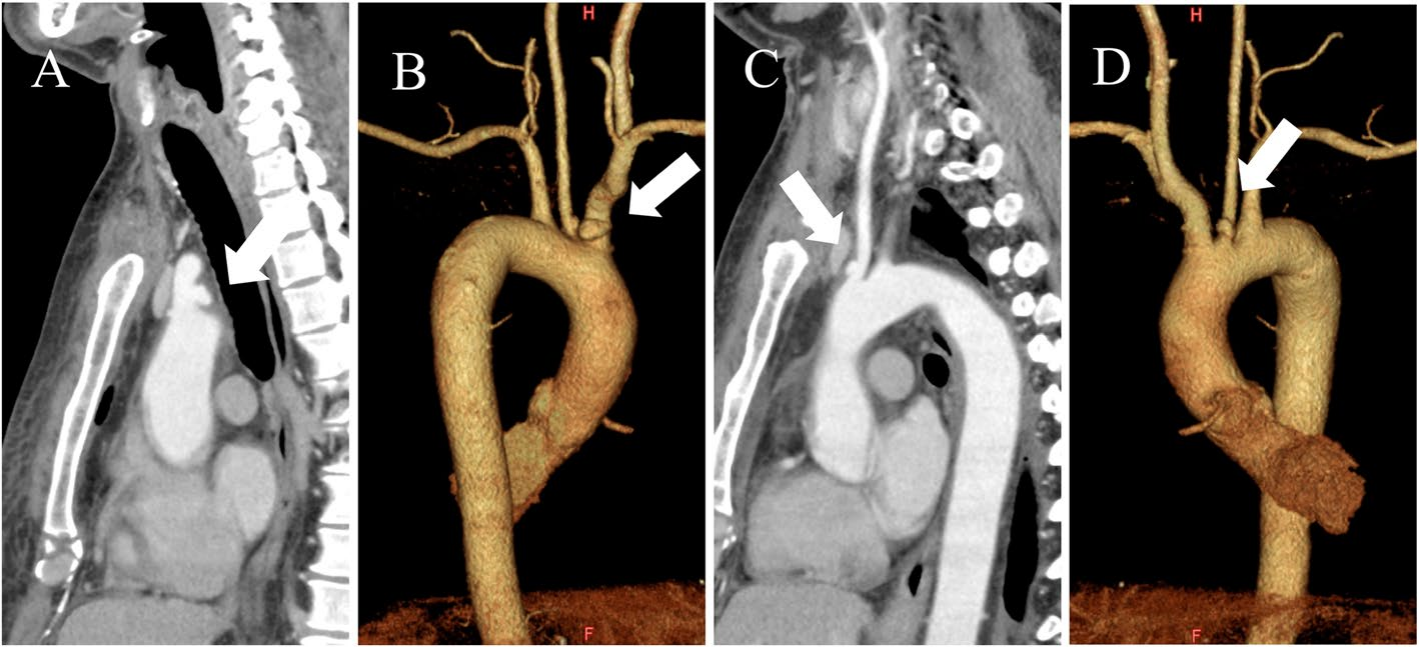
CT scans 2 weeks later following emergency admission. An enlargement of the BCA pseudoaneurysm (white arrows) was detected in a sagittal slice of CT (A) and a three-dimension CT (B). A newly developed LCA pseudoaneurysm (white arrows) was detected in a sagittal slice of CT (C) and a three-dimension CT (D)
